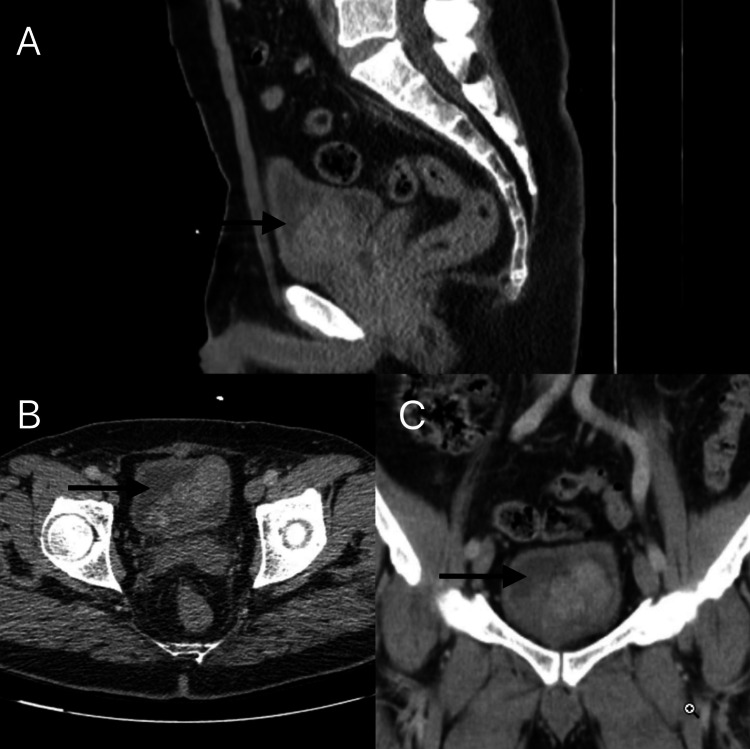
Contrast-enhanced CT of the mass Sagittal (A), axial (B), and coronal (C) slices showing a mass in the bladder lumen (black arrows).
